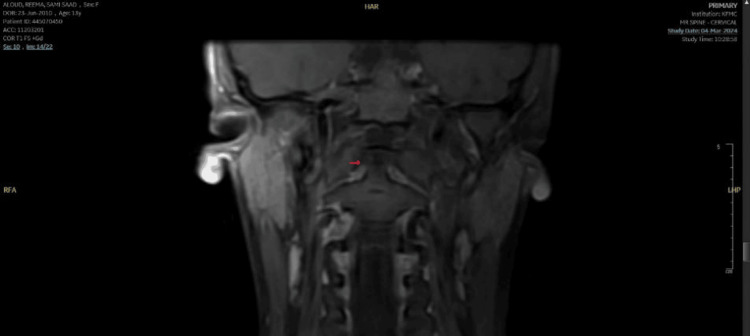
MRI coronal view of a cervical spine showing resolution of AAS AAS: atlantoaxial dislocation
